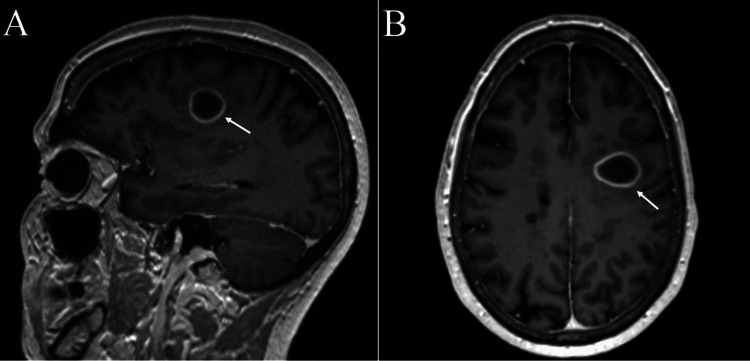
MRI brain Magnetic resonance imaging (MRI) of the brain with T1 post-contrast sagittal (A) and axial (B) slices demonstrating a 2.1 cm x 2.6 cm x 1.9 cm ring-enhancing lesion within the left frontal centrum semiovale superior to the frontal operculum, as indicated by the white arrows.
